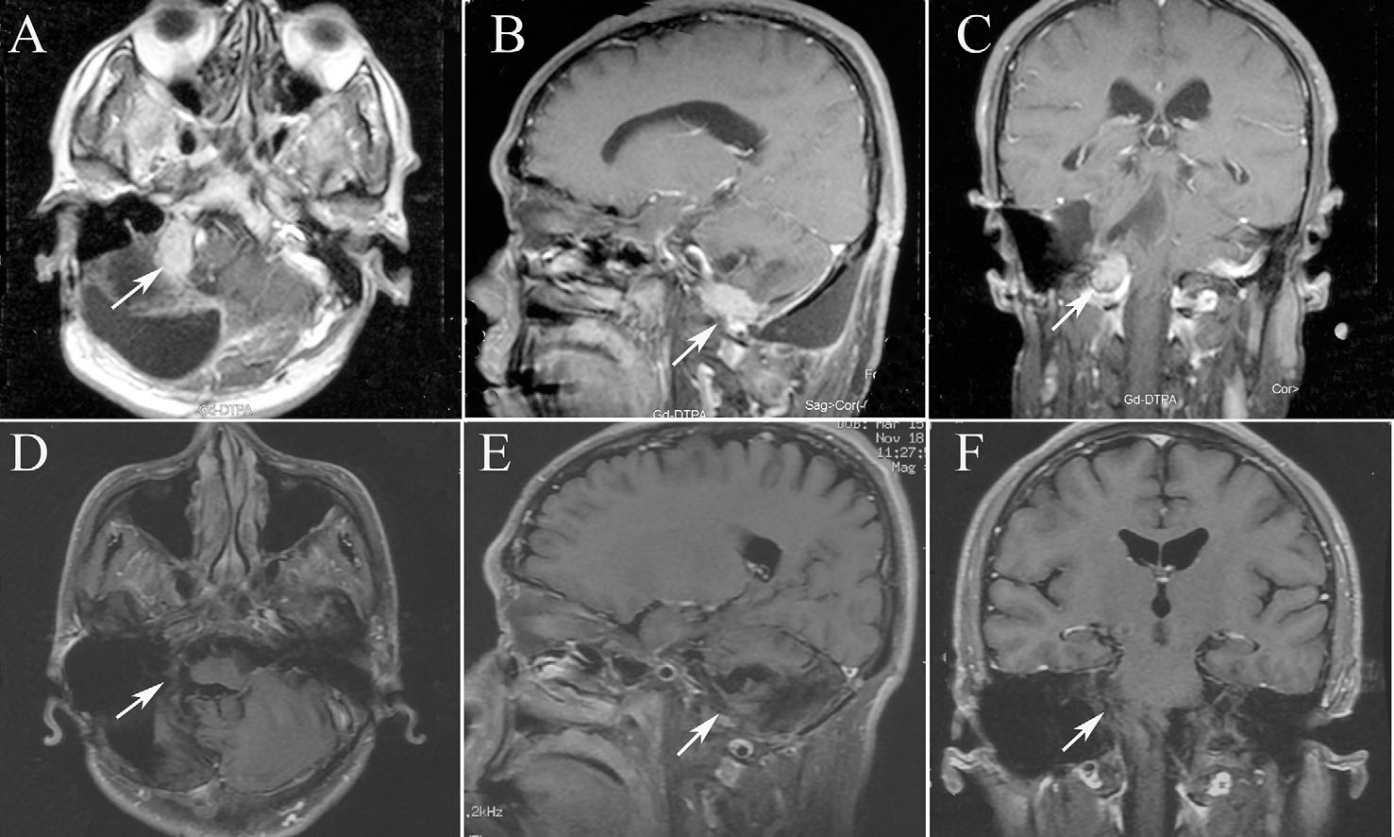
The MRI images of patient 7, tumor 16. (A) Axial T1-weighted contrast-enhanced image showing residual enhancement and a pretreatment tumor volume of 11.33 cm^3^ (tumor 16); (B) Sagittal T1-weighted contrast-enhanced image; (C) Coronal T1-weighted contrast-enhanced image; (D) Axial T1-weighted contrast-enhanced image after 13 months, the tumor disappeared. (E) Sagittal T1-weighted contrast-enhanced image after 13 months; (F) Coronal T1-weighted contrast-enhanced image after 13 months.
